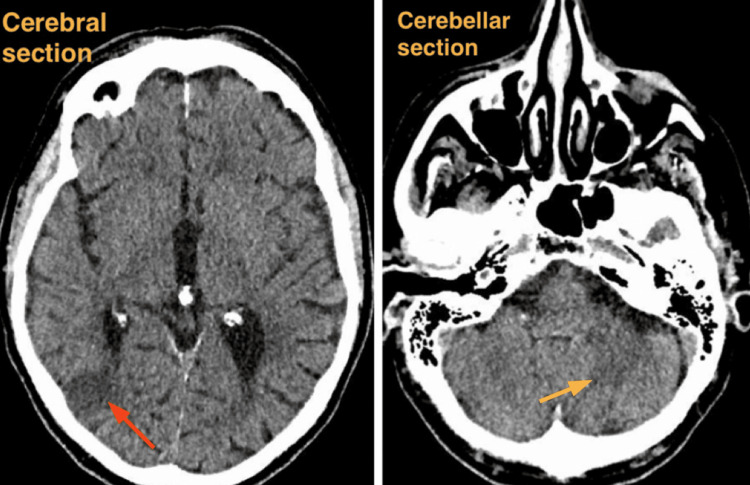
Non-contrast CT head on admission. Cerebral section on the left, and cerebellar section on the right On the left, cerebral section showing right temporal-occipital lesion. On the right, cerebellar section showing smaller lesions in the right cerebellar hemisphere and an ill-defined area of low density in the left cerebellar hemisphere. The red arrow is pointing to the site of cerebral lesion in the right temporal-occipital region. The yellow arrow is pointing to the site of the left cerebellar hemisphere lesion.
